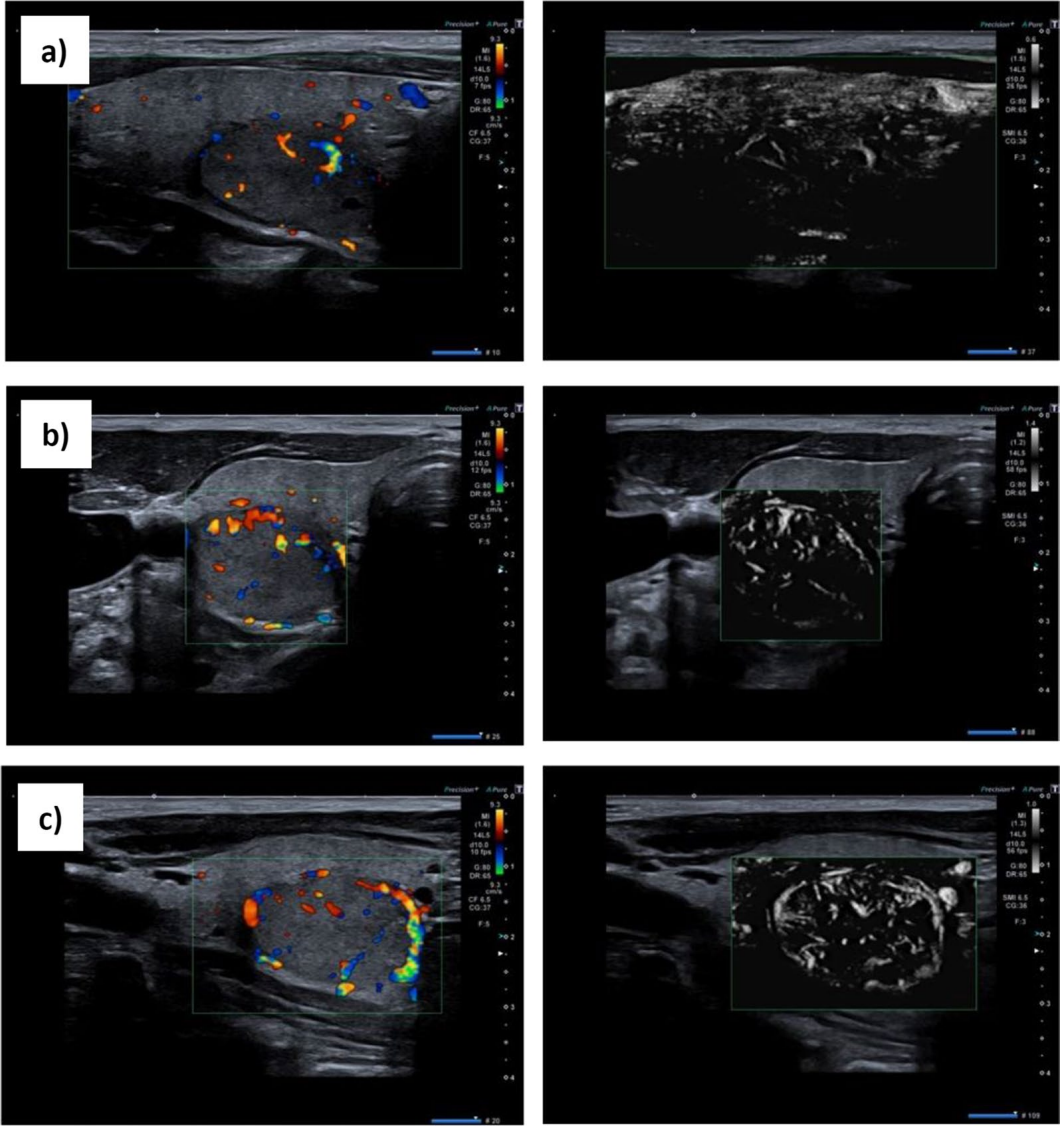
User interface of a Toshiba Aplio i800 ultrasound imaging system. (Left panels) Doppler sonogram displayed in colour Doppler mode; (right panels) the same sonogram displayed in monochromatic SMI mode. a Creation of the ROI total (green rectangle); b creation of the ROI-A (green square) in the cross-sectional view and c ROI-A (green rectangle) in the longitudinal view
